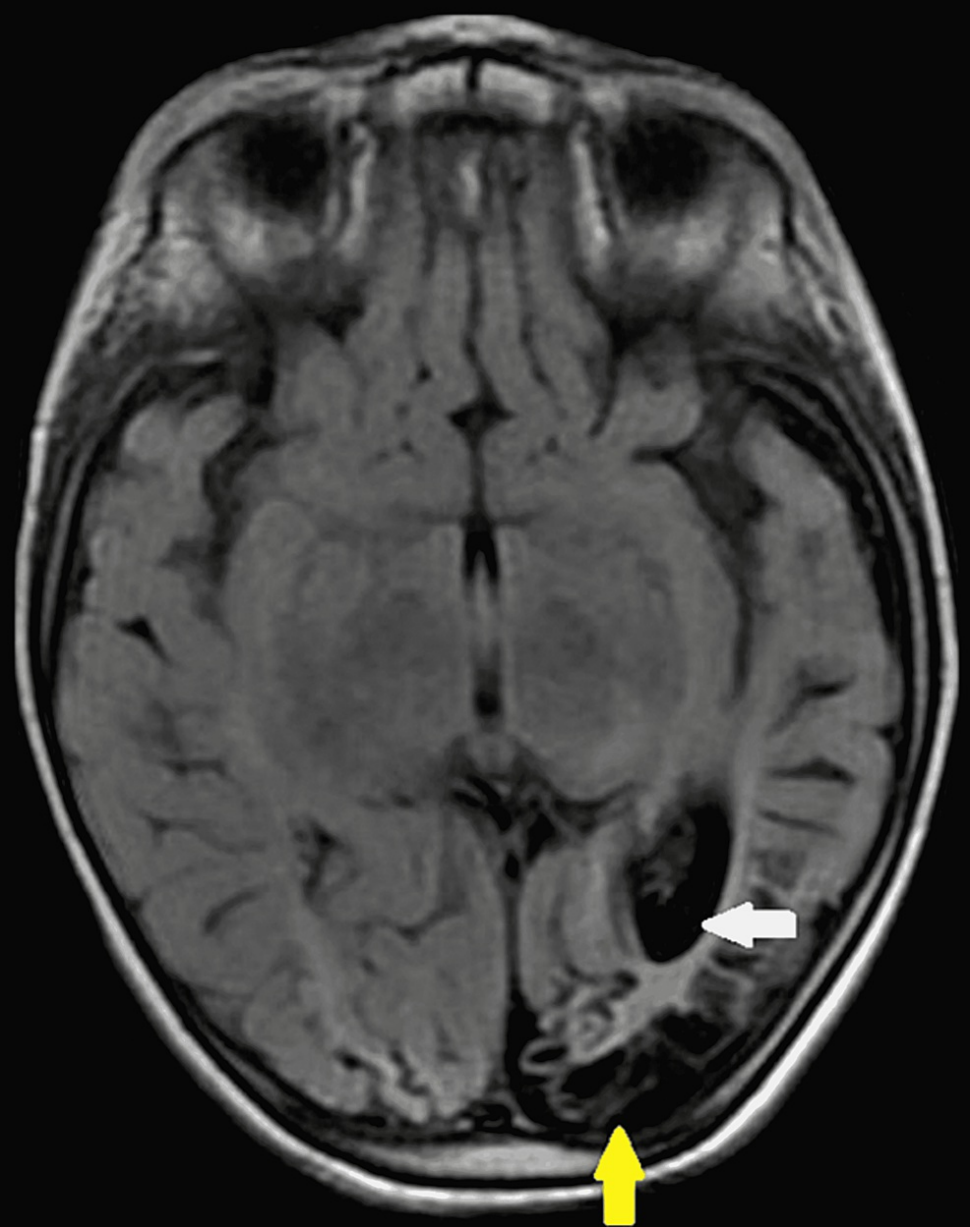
Axial T2 FLAIR image of a two-year-old child with developmental delay showing cystic encephalomalacia with adjacent gliosis (yellow arrow), volume loss, and ex-vacuo dilatation of the  occipital horn of the left lateral ventricle (white arrow).
